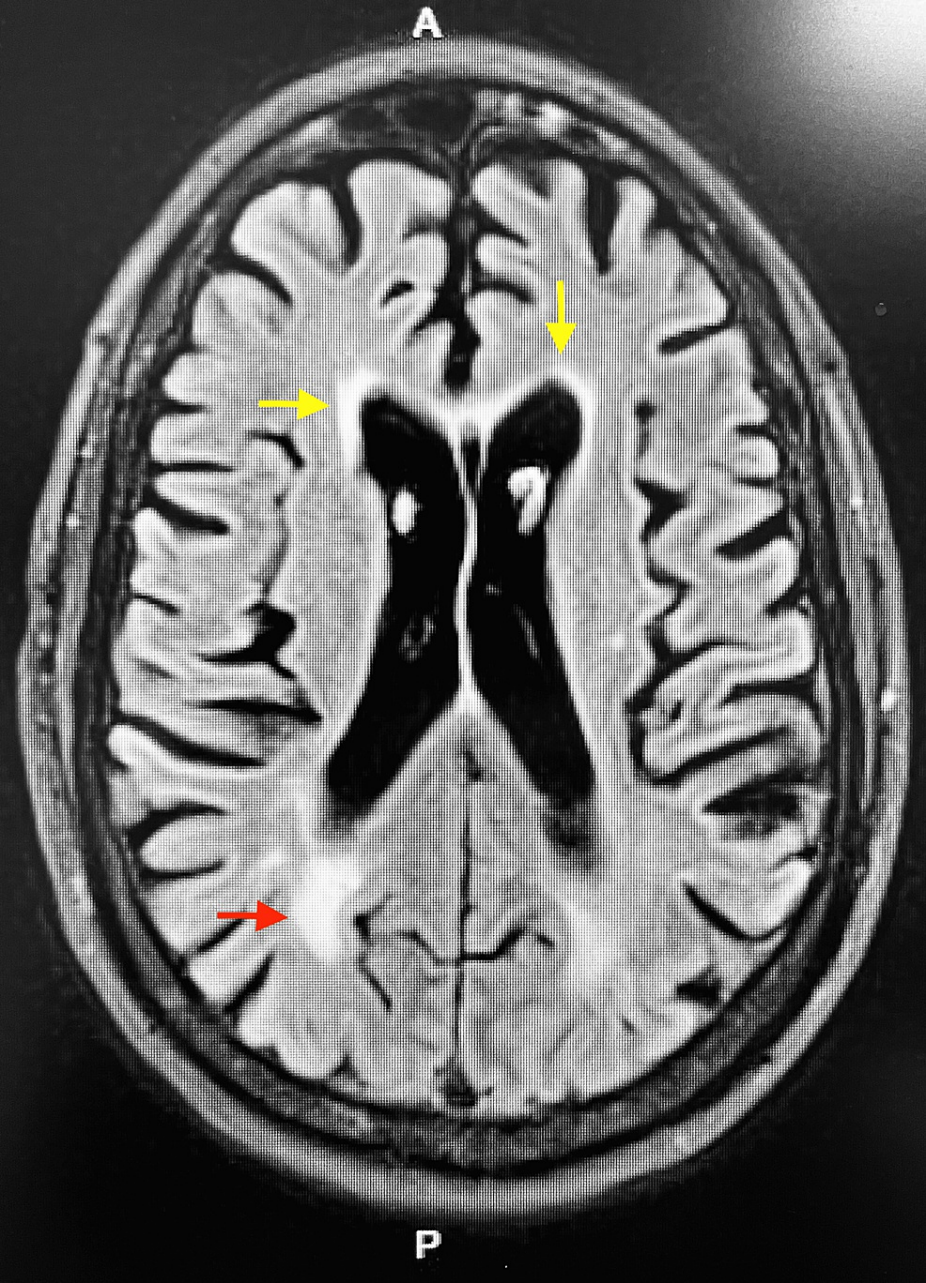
MRI Brain showing increased T2 signal within the periventricular (yellow arrows) and subcortical (red arrow) white matter; characteristic of toxic leukoencephalopathy.
